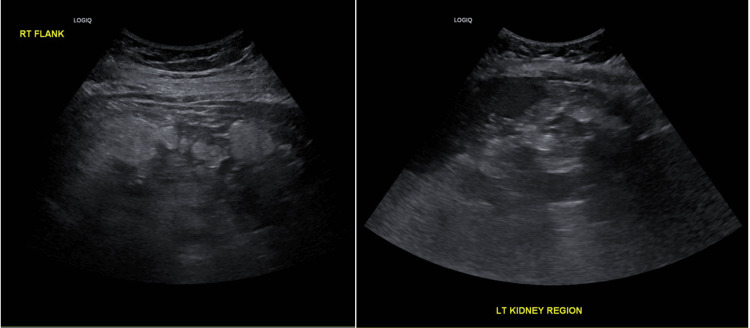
US abdomen: views of right and left kidney regions Ultrasound (US) abdomen images show large echogenic fat deposits across the kidney regions.
